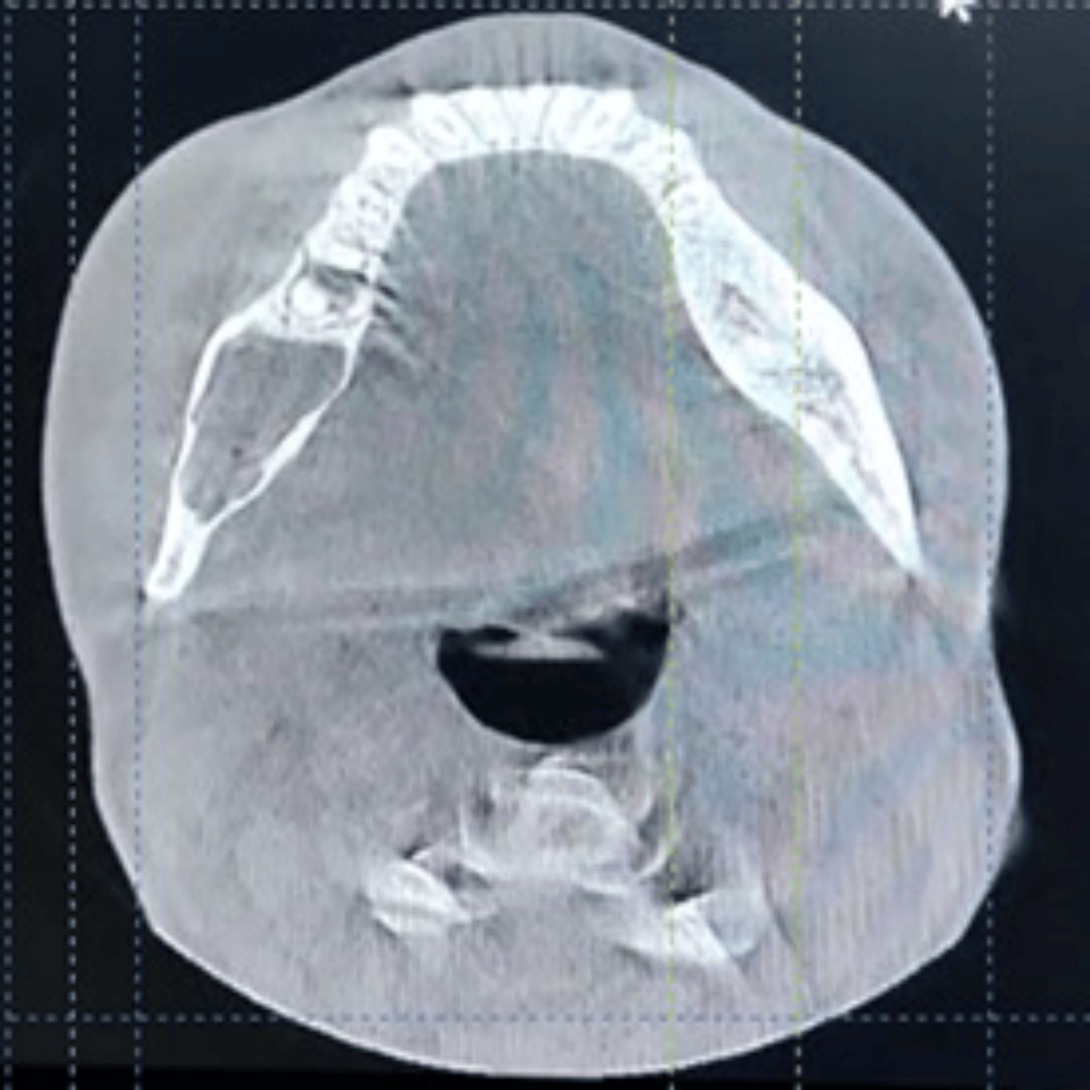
Computed tomography (CT) demonstrated expansion and thinning of the buccal and lingual cortical plates, with thinning and perforation of the medial surface of the ramus of the mandible
